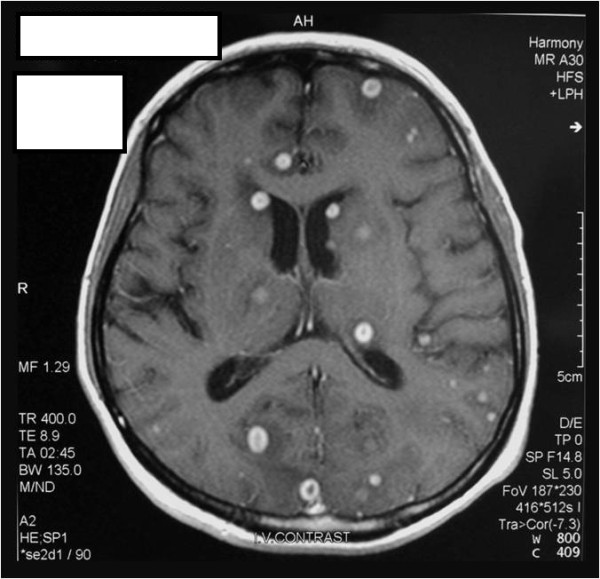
A magnetic resonance image with gadolinium enhancement at initial presentation. The image shows multiple ring-enhancing lesions spread diffusely throughout the brain.
